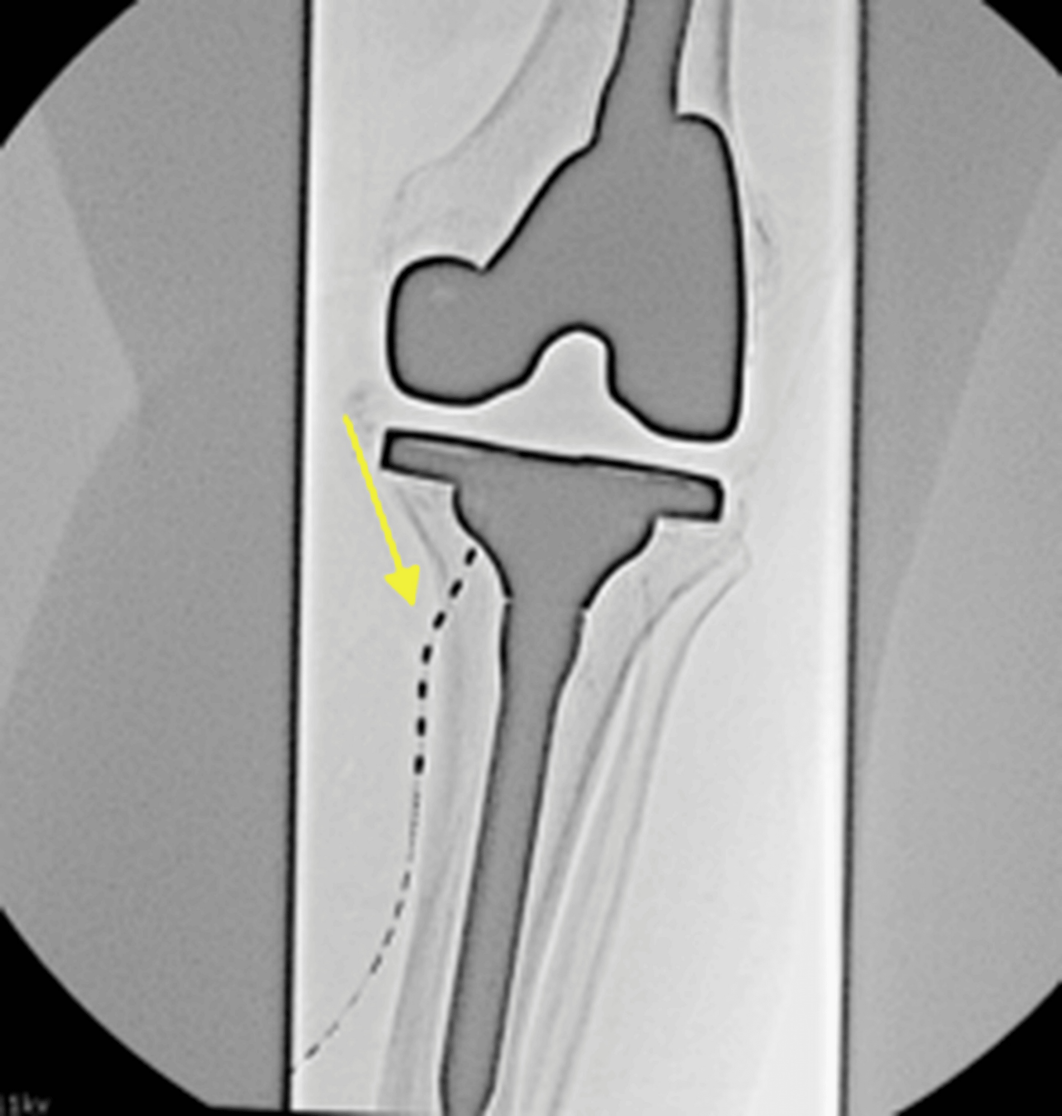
Anteroposterior radiograph of the peripheral nerve stimulator (PNS) trial targeting the left inferomedial genicular nerve. The lead (indicated by the yellow arrow) is positioned along the tibial midshaft and flare.
